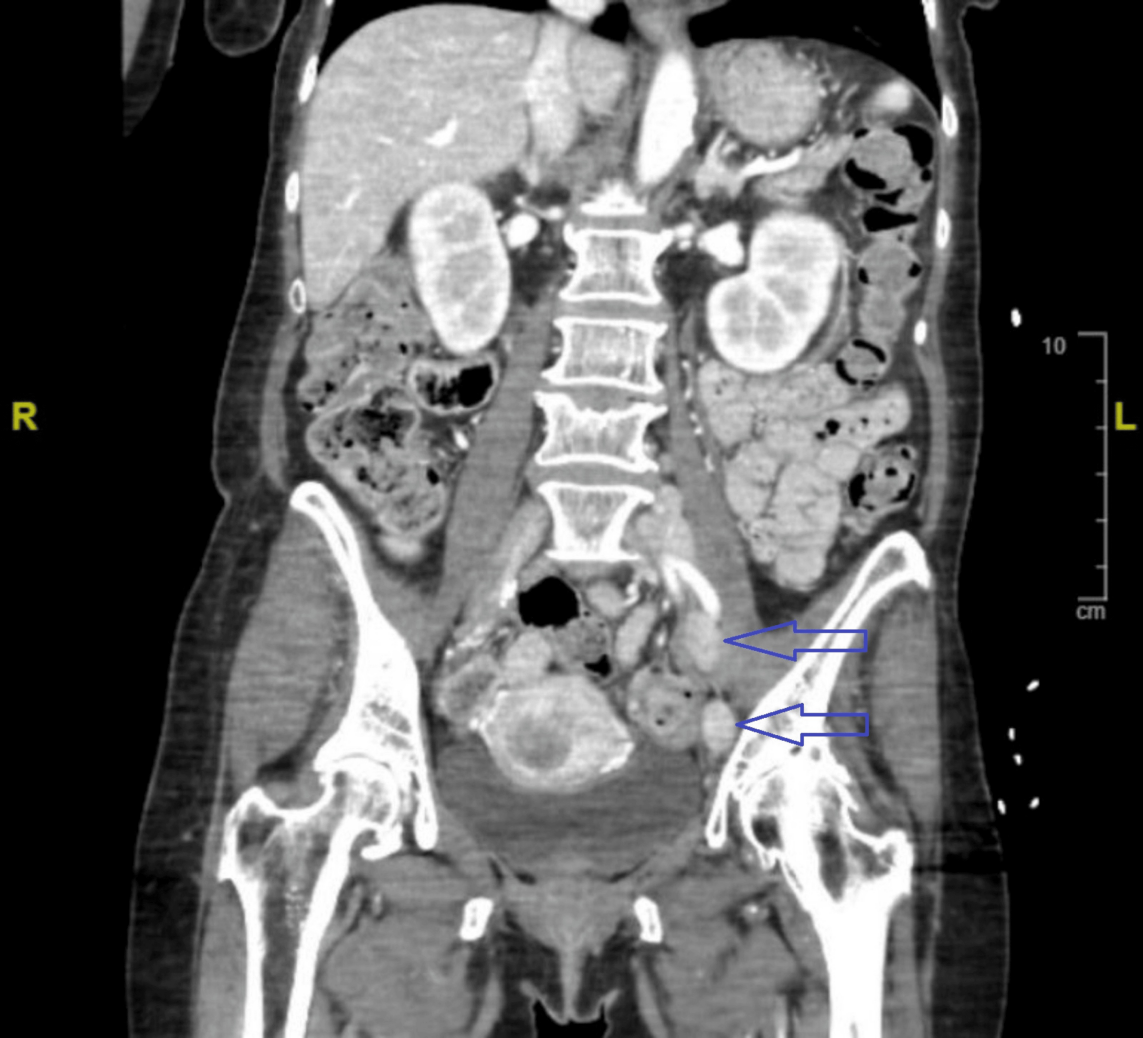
Computed tomography of the abdomen and pelvis demonstrating left inguinal chain lymphadenopathy with blue arrows pointing to the inguinal chain lymphadenopathy
